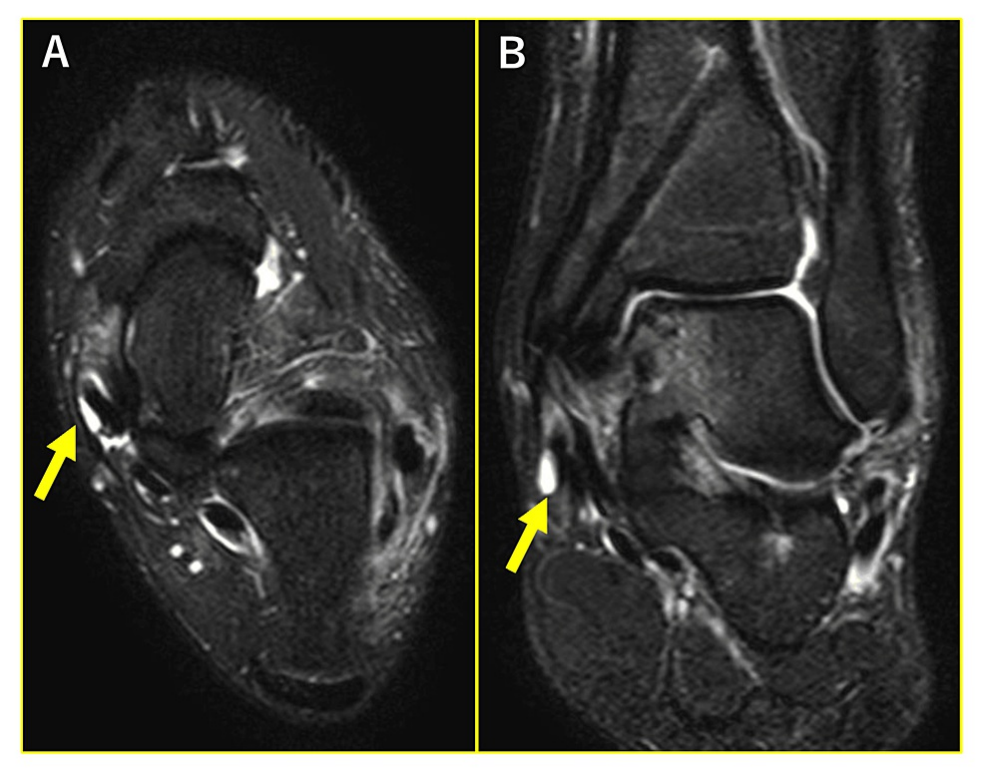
Representative images of posterior tibial tendon (PTT) abnormalities on preoperative and one-year postoperative MRI. (A) Preoperative MRI. (B) One-year postoperative MRI. Arrows indicate PTT abnormality.
